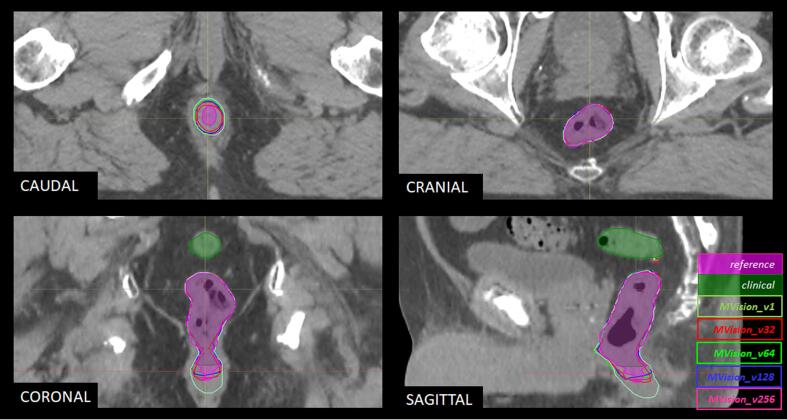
Example outputs from original and retrained versions of the MVision deep-learning algorithm on planning-CT images for one prostate cancer case. Note how the original version of the autosegmentation algorithm (MVision_v1; colour coding in image) results in a volume similar to the reference curated volume (filled purple) in the cranial direction but below the reference in the caudal direction and with a somewhat larger circumference. The recalibrated versions (MVision_v32-256; colour coding in image) remain at the same anatomical border as the original version but approach the lower anatomical border and the circumference of the reference. The anatomical border of the clinical uncurated volume (filled green) is above the reference in the cranial direction. (For interpretation of the references to colour in this figure legend, the reader is referred to the web version of this article.)
